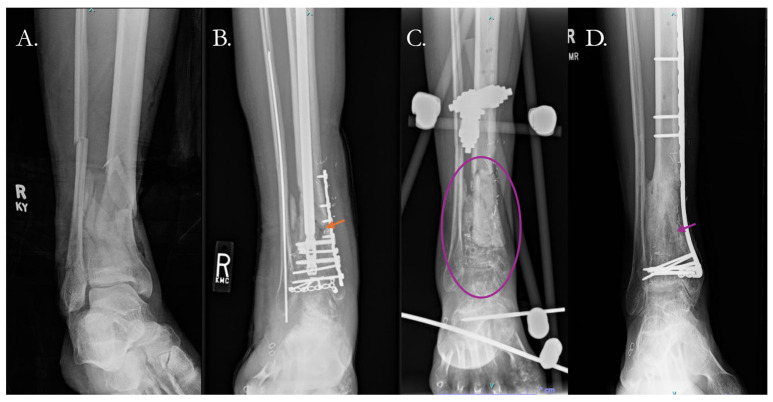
Nonunion of pilon fracture with induced membrane and local biologic therapy. 27-year-old male who was in a motor vehicle accident resulting in a pilon fracture. One year after his injury, the patient was diagnosed with septic tibial nonunion and underwent repair with an induced membrane, iliac autograft and rhBMP-2. (A) Radiograph of initial injury demonstrating comminuted distal tibial fracture with intra-articular extension and ipsilateral fibula fracture. (B) Radiograph of primary hardware at over 6 months after initial fixation when the patient was diagnosed with infected nonunion. Orange arrow pointing at persistent fracture lines. (C) Radiograph of antibiotic spacer (purple oval) resulting in an induced membrane (not visible) with external fixator in place to hold alignment. (D) Radiograph 3 years following repair with evidence of fracture consolidation (purple arrow) and new plate fixation.
